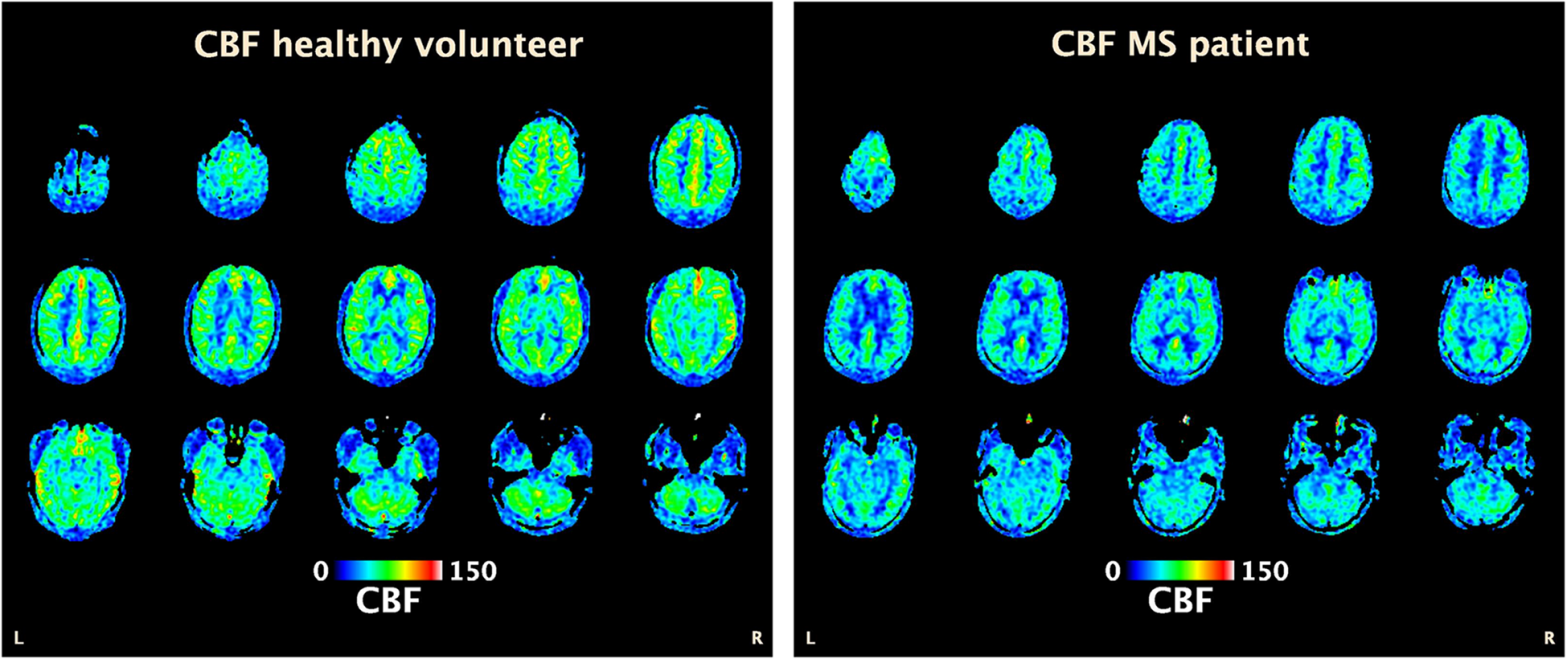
Brain perfusion is globally decreased in MS. Perfusion-weighted brain MRI (arterial spin labelling) from a 35-year old healthy male volunteer (left) versus a 34-year old man with relapsing-remitting MS (right), with CBF color-coded map overlay. Brain perfusion is globally reduced in the MS patients, as compared to the healthy volunteer. CBF: cerebral blood flow (expressed as mL/100 g/min), MS: multiple sclerosis, MRI: magnetic resonance imaging
